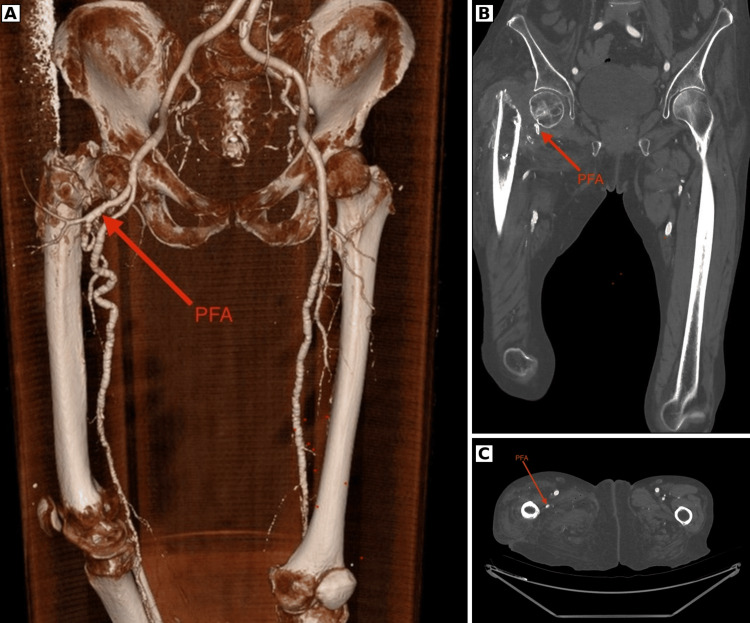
Pre-operative CT angiography demonstrating close relation of the profunda femoral artery to the proximal femur Pre-operative CT angiographic imaging demonstrating the vascular anatomy on the affected side. (A) 3D reconstruction showing the profunda femoral artery in close relation to the proximal femur. (B) Coronal image demonstrating the vessel adjacent to the medial proximal femur. (C) Axial image showing the vessel in close proximity to the proximal femur, supporting pre-operative planning. PFA, profunda femoral artery.
